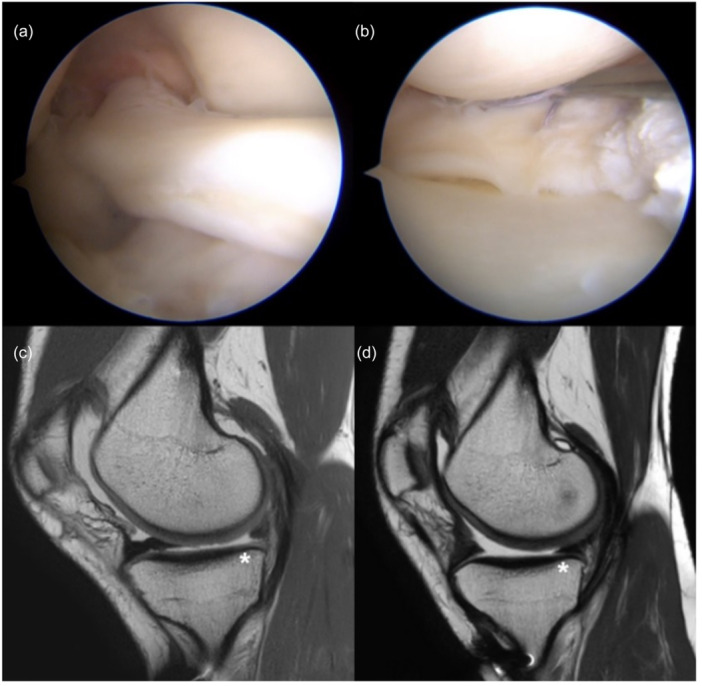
Medial bucket handle repair. Arthroscopic and MRI images of a medial bucket‐handle meniscal tear (a, c), repaired using the all‐inside technique (b). Postoperative follow‐up MRI demonstrates complete healing (d). MRI, magnetic resonance imaging.
